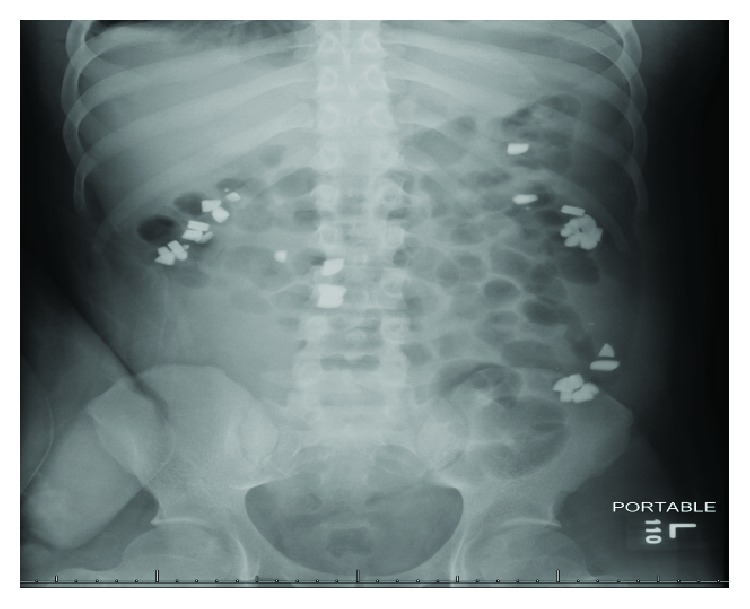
An abdominal radiograph from 6/26/18 showing multiple radiopaque objects in the bowel.
